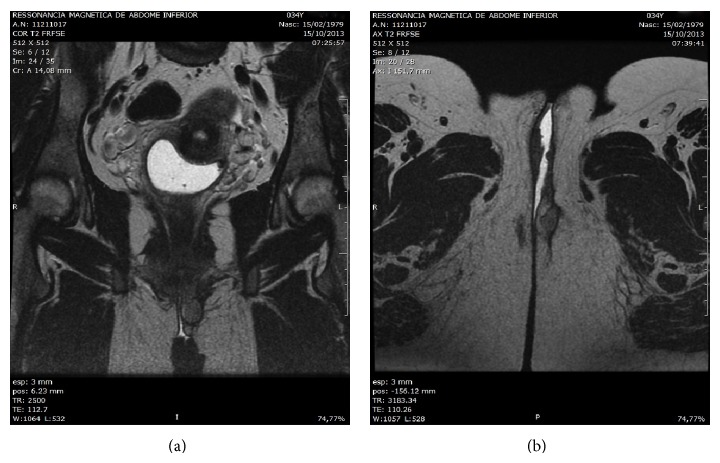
(a) Coronal and (b) axial MRI images with a 2.4 × 2 × 1.4 cm size soft tissue mass, in the left labia majora, and no pelvic and inguinal lymphadenopathy.
